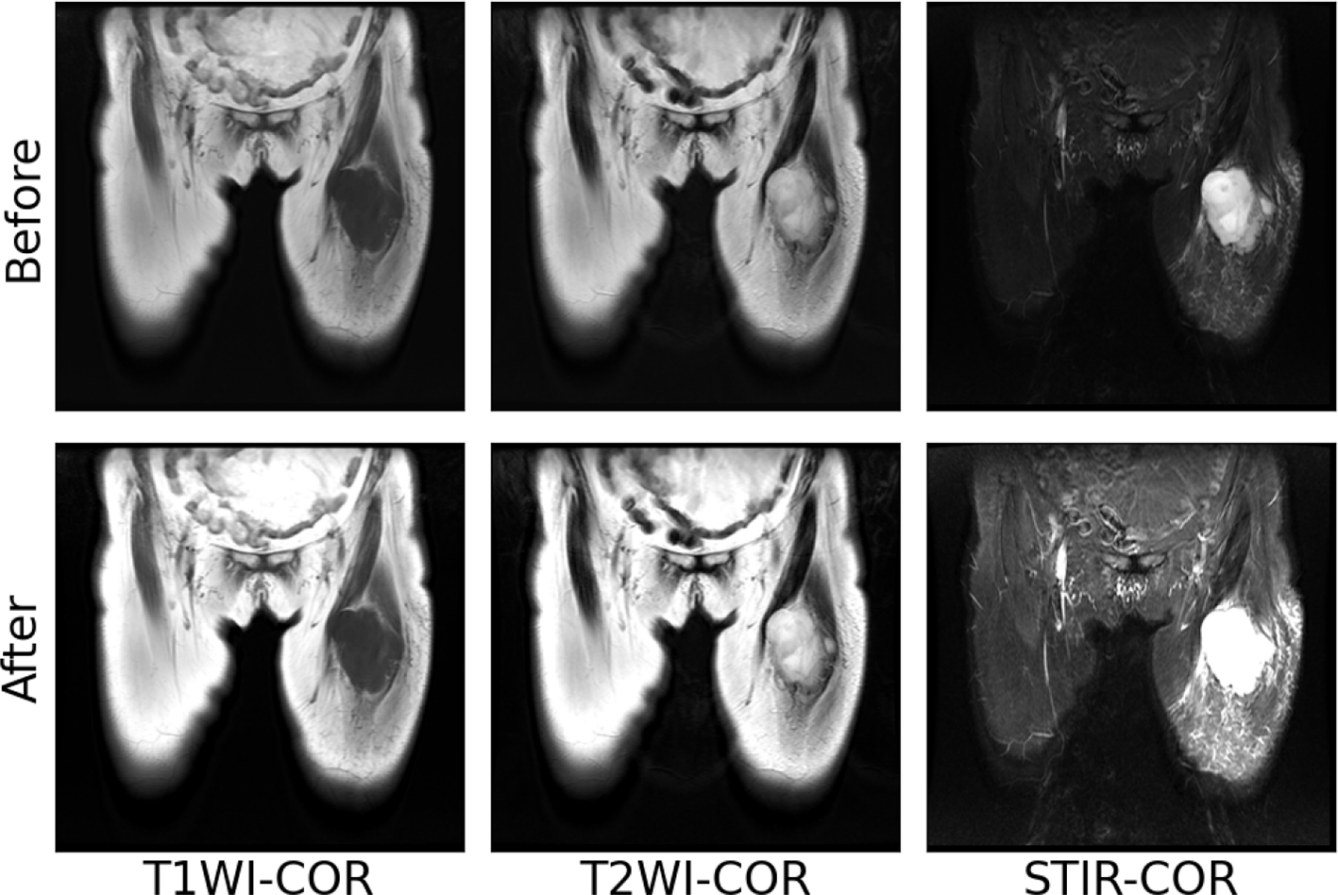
Maximum–minimum normalized pre-post images of individual modal sequences of MRI in patients with soft tissue sarcoma.
